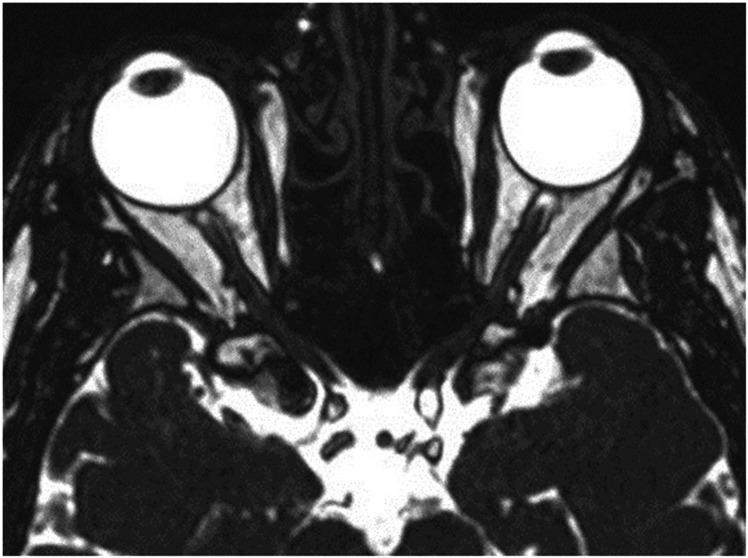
MRI images of a 71-year-old male control without kinking of the optic nerve. No kink visible in the axial plane.
